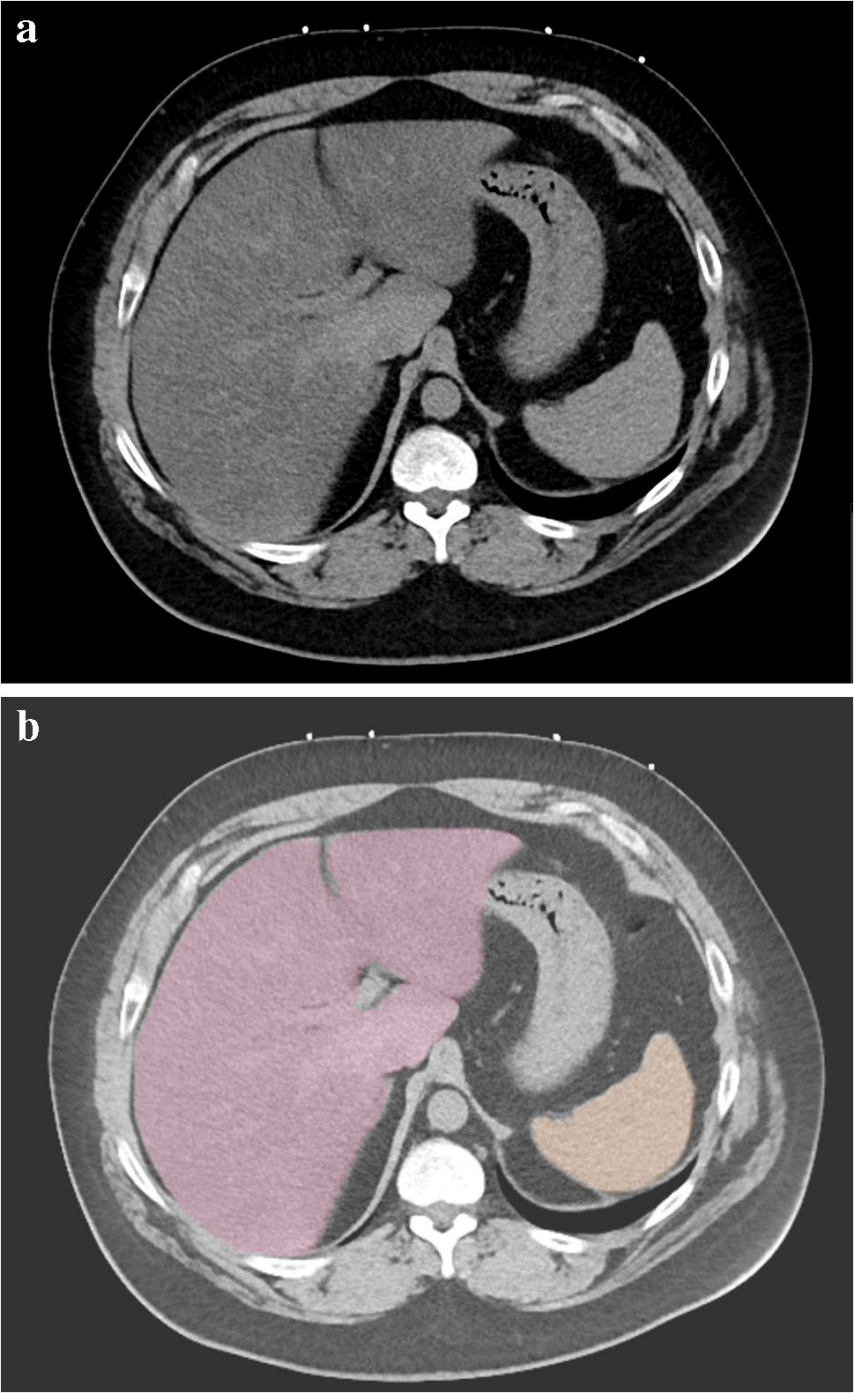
A Abdominal CT image of patient with hepatic steatosis. AI analysis yielded a liver volume of 3010 mL with a mean attenuation of 25 HU; mean attenuation of the spleen was 47 HU. B Corresponding AI-processed image includes color overlays to highlight the segmentation of the liver (pink) and spleen (yellow)
